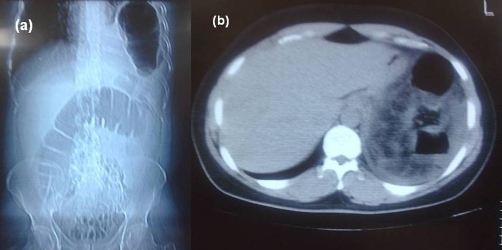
(a) X-ray of the abdomen and thorax showed multiple air-filled structures in the left hemithoracic area, widening of the intercostal space and displacement of the mediastinum to the right. Notice the continuity of the colonic gas shadow that can be clearly seen on abdomen and left hemithorax. (b) Abdominal CT section showed a thickened posterior diaphragm. Colonic segments can be seen just anterior to the diaphragm.
